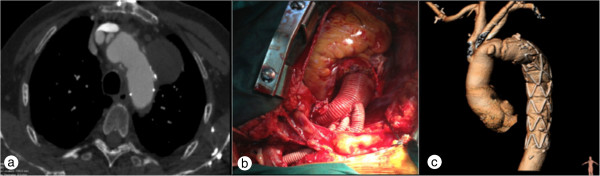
Intra-operative photographs of surgical repair and post-operative imaging. (a) CT angiogram axial view demonstrating postoperatively that the pseudo-aneurysm has been thrombosed; (b) Intra-operative photograph demonstrating total aortic arch replacement and anastomosis with head and neck vessels; (c) 3D-reconstucted CT angiogram demonstrating the frozen elephant trunk and patency of anastomoses of head and neck vessels.
